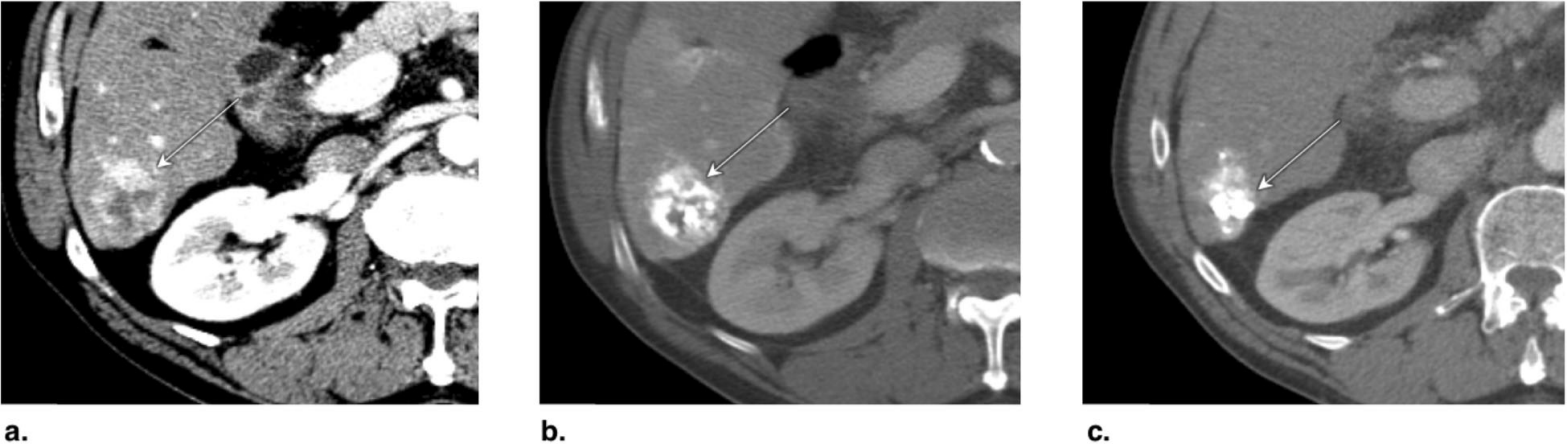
Axial CT obtained (a) pretreatment: image in late arterial phase (AP) shows nonrim arterial phase hyperenhancement of a 3.5-cm LR-5 observation in segment VI (arrow). b 1 month post transarterial chemoembolization (TACE): image in portal venous phase shows ethiodized oil retention with optimal targeting (arrow). c 2 months post TACE: image in late AP shows decrease of ethiodized oil retention (arrow). Density of ethiodized oil limits the assessment at the anterior part of the lesion. The treated observation is categorized LR-TR Equivocal
